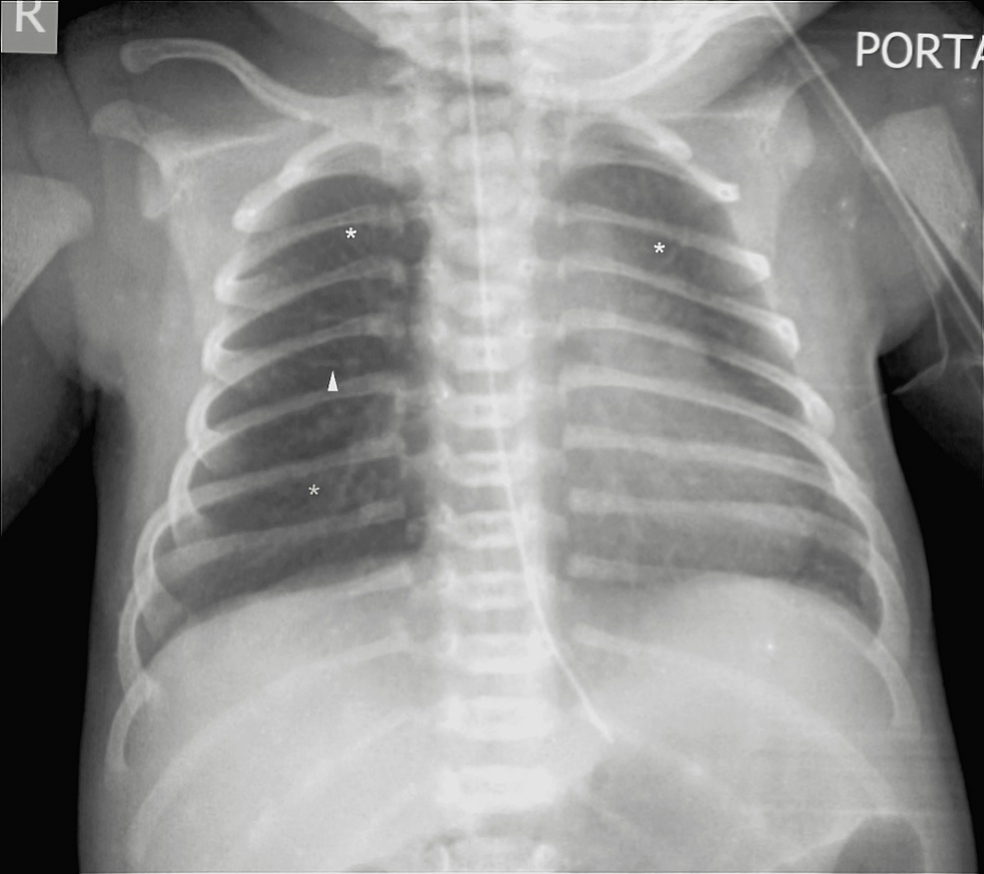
Chest radiograph in transient tachypnea of the newborn The radiograph shows typical features of TTN. Straightened ribs and increased intercostal space due to mild hyperinflation lungs. streaky infiltrates (white asterisks), fluid in horizontal lung fissures (white arrow), perihilar streaking (Sunburst)
